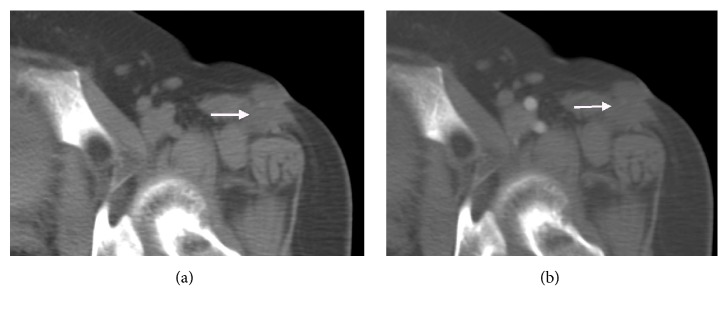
Images obtained from a 55-year-old woman with EMC of the left thigh. (a, b) Transverse CT scan showed low density, round mass without marked enhancement (white arrow).
